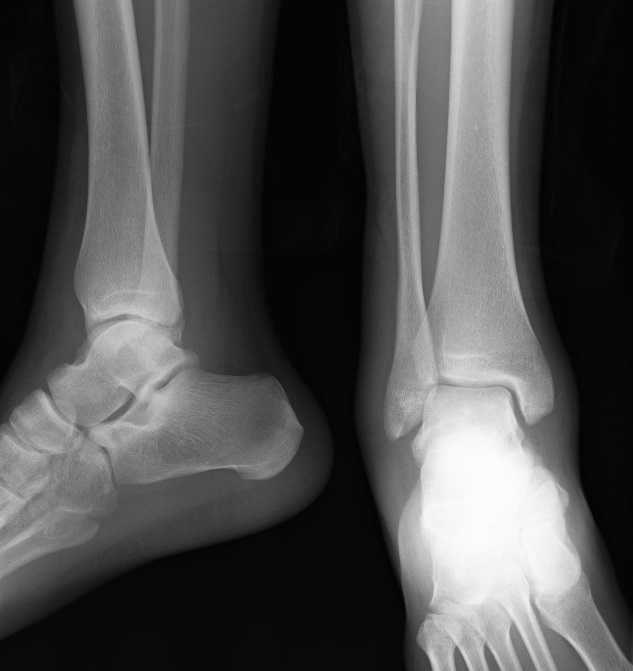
Radiographies de la cheville face et profil après réduction confirmant l'absence de fractures
